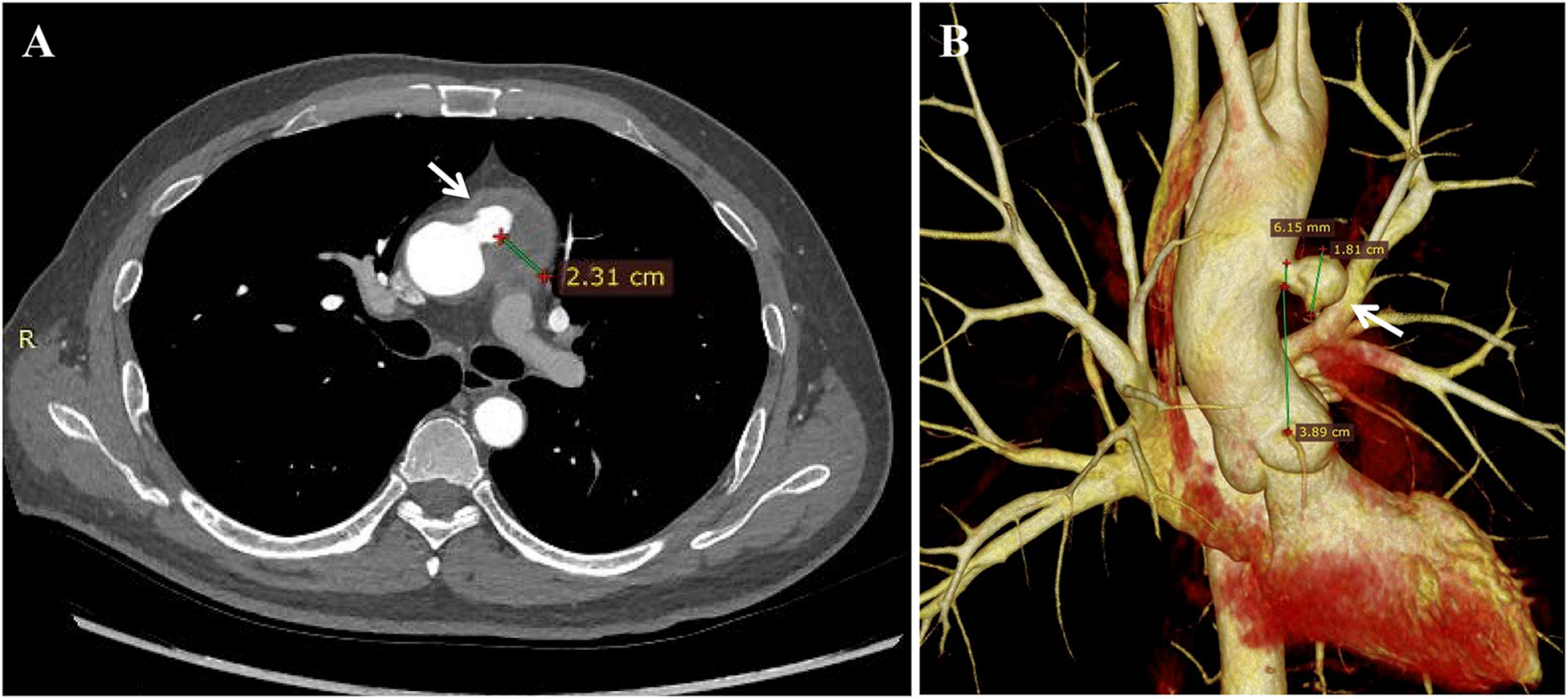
Preoperative CT angiography revealed (A) the pseudoaneurysm located at the anterior wall of the ascending aorta (indicated by the white arrow), with an adhered thrombus of maximum thickness 23 mm observed on its external surface. (B) Morphological measurements of the lesion were obtained from 3D reconstructed images, showing a maximum pseudoaneurysm neck diameter of 6 mm, maximum pseudoaneurysm sac diameter of 18 mm, and a distance of 39 mm from the aortic sinus to the pseudoaneurysm.
